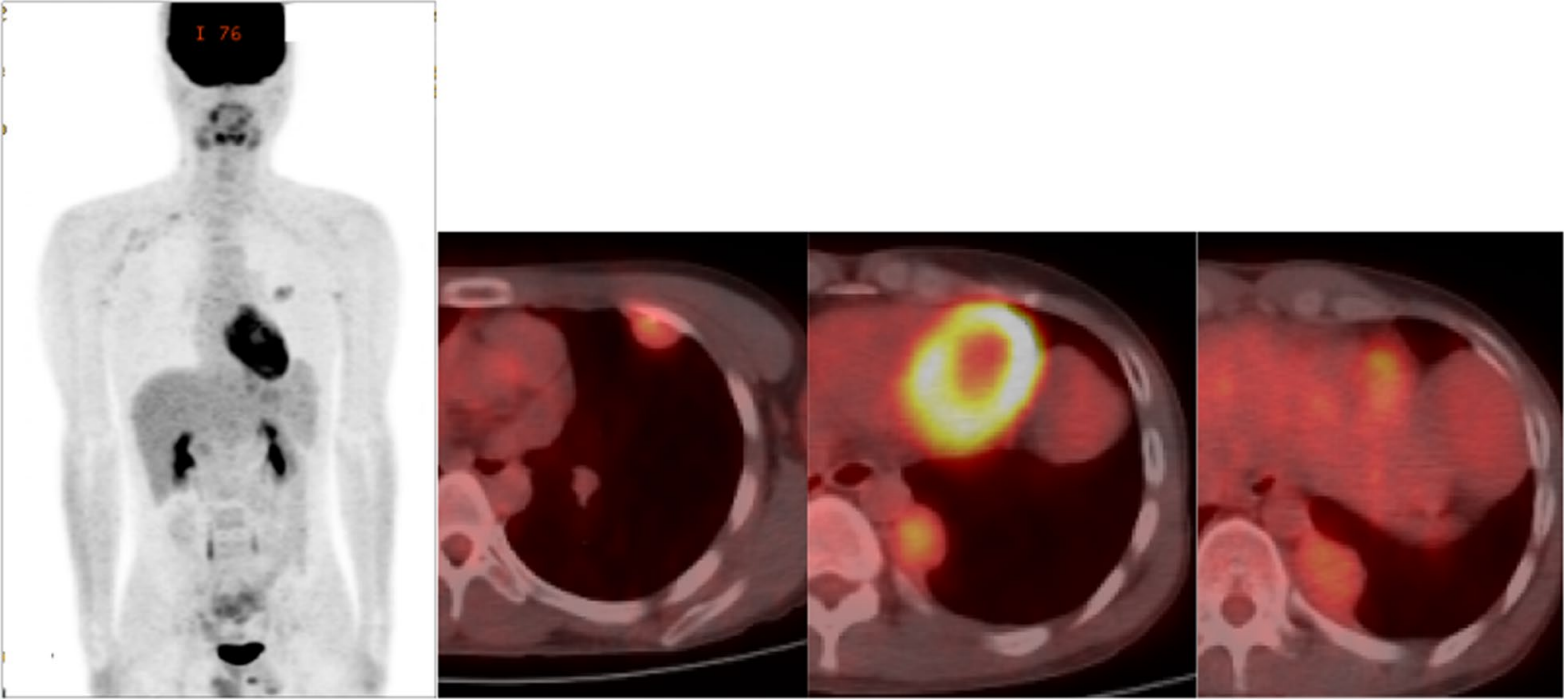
FDG-PET showed an SUVmax of 0.5 to 3.2 within each tumor
